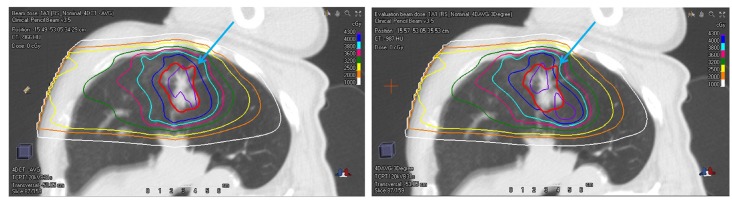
An individual beam dose distribution of the nominal plan on the planning CT (left) and three-degree counter clock-wisely rotated planning CT (right). The thick red line denotes the iGTV. CT: Computed tomography; iGTV: internal gross target volume.
